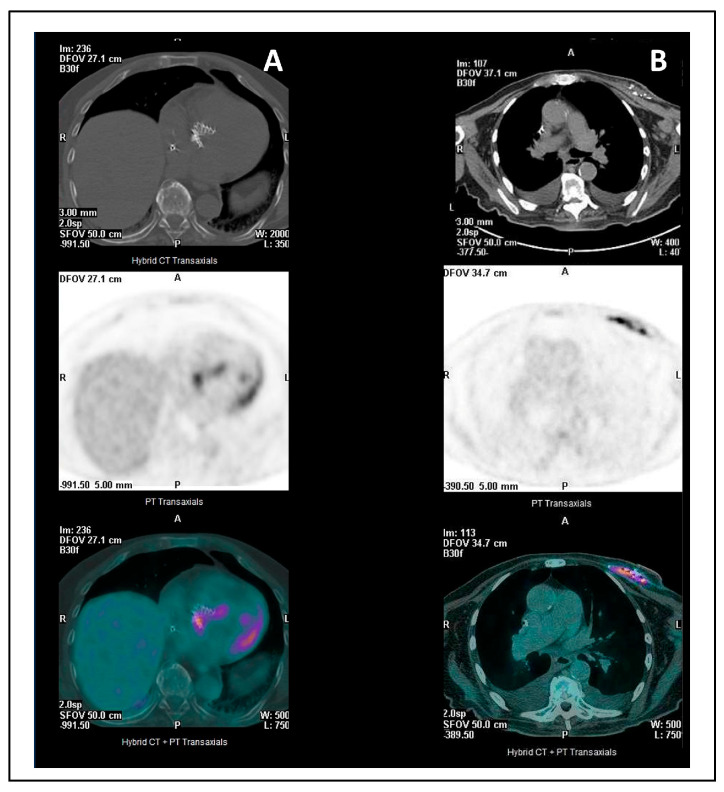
FDG-PET/CT findings in prosthetic valve endocarditis and cardiac device-related infective endocarditis. Panel (A). Prosthetic valve endocarditis with perivalvular abscess. From top to bottom: Non-contrast-enhanced CT (low-dose CT for attenuation correction and anatomical localization) showing a biological aortic valve prosthesis with a perivalvular low-attenuation collection consistent with abscess formation. Attenuation-corrected PET demonstrating focal increased FDG uptake in the periprosthetic region. Fused PET/CT images confirming intense, focal FDG uptake spatially corresponding to the perivalvular collection, consistent with metabolically active infection. Panel (B). Cardiac implantable electronic device pocket infection. From top to bottom: Non-contrast-enhanced CT showing soft tissue thickening at the generator pocket site. Attenuation-corrected PET demonstrating focal FDG uptake at the device pocket. Fused PET/CT images confirming localized increased FDG uptake at the pacemaker pocket, consistent with active device-related infection. These examples highlight the complementary value of anatomical CT and metabolic PET imaging for the detection of intracardiac and extracardiac infectious complications, particularly in prosthetic valve endocarditis and cardiac device-related infective endocarditis.
